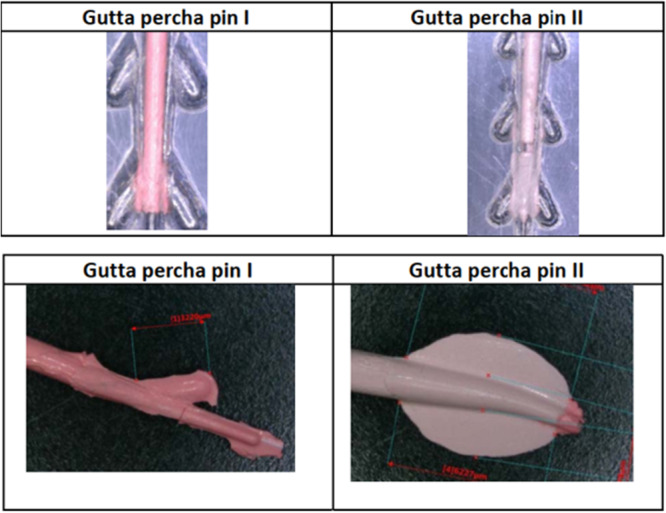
Melted gutta‐percha flowing sideways, comparable to lateral canals, after ultrasonic instrumentation.
